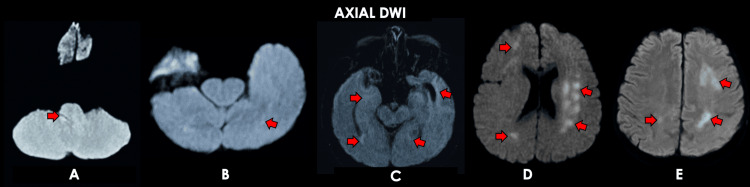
Representative cranial MRI sequences for patient #1. Representative axial brain MRI (DWI sequence) images show restricted diffusion at the sites of the previously described FLAIR-hyperintense lesions in Figure 1, including the right lateral medullary area (A); bilateral temporal and occipital areas (B, C); the left frontoparietal area; right frontal and parietal areas; the left corona radiata; and bilateral centrum semiovale (D, E). FLAIR: Fluid-attenuated inversion recovery; DWI: diffusion-weighted imaging.
